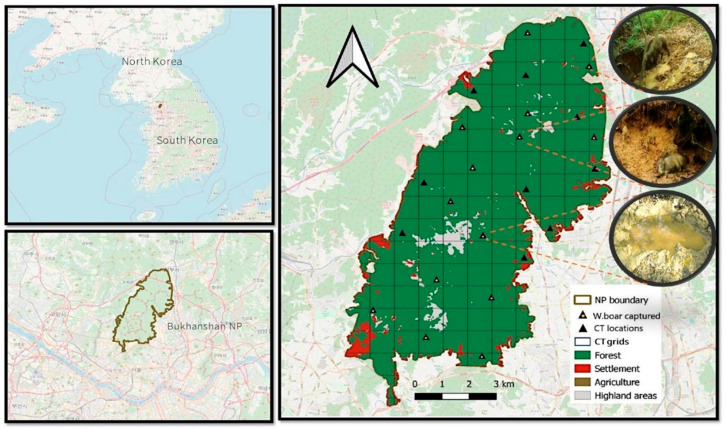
Map of the study area and camera trap (CT) locations within Bukhansan National Park, South Korea. The images on the right (within the circle) depict puddles used for wallowing by wild boars at various CT locations. The white arrow indicates the north.
